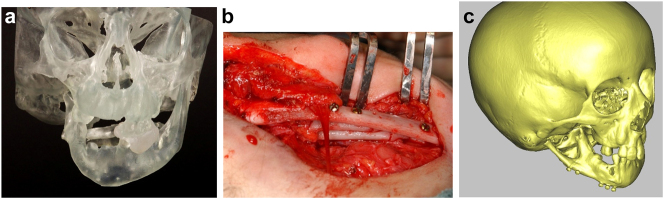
Construction of mandible with rib grafts. (a–c) A PK IIb case with long costo-chondral graft and further rib grafts to fill the defect with CT outcome.
